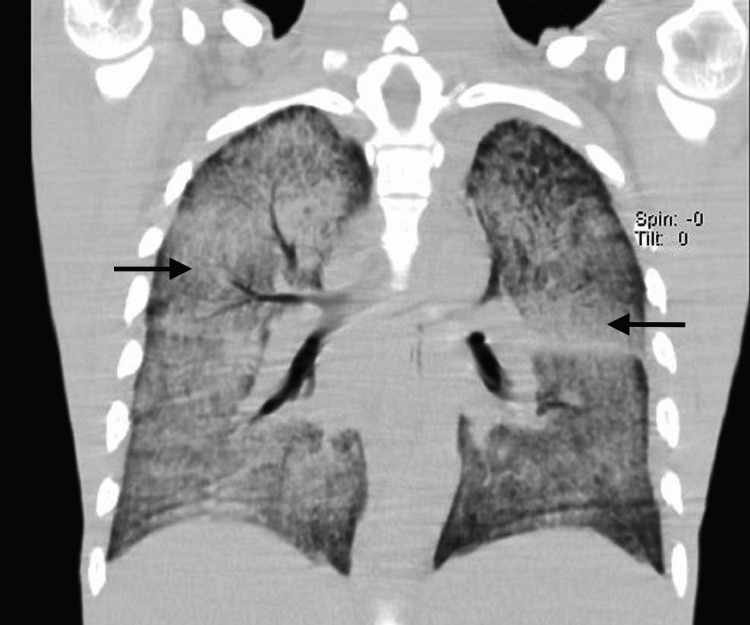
Coronal view of the thorax computed tomography scan To further investigate the alterations on the previous X-ray, a computed tomography scan was performed. As demonstrated, a severe bilateral consolidation of both lungs is present (arrows).
